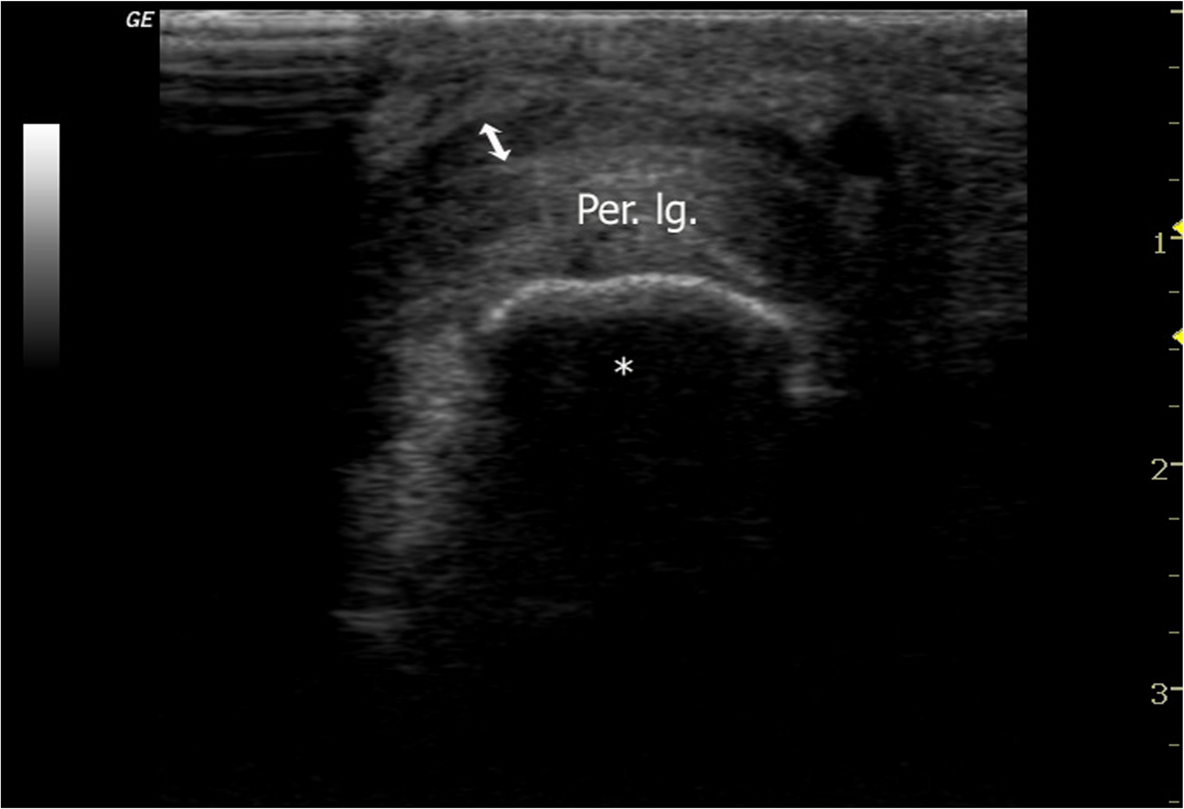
Transverse section ultrasonography of the mass over the peroneal tubercle. An enlarged peroneus longus tendon covers the peroneal tubercle. * = peroneal tubercle. Per. lg. = dislocated peroneus longus tendon. Double arrow = effusion in the peroneus longus tendon sheath
